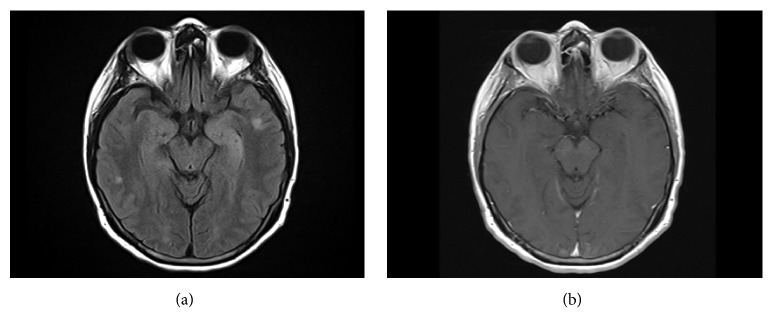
MRI brain imaging for Case*    *2. (a) MRI brain FLAIR imaging. This image demonstrates mildly expansile T2 signal hyperintensity of the left greater than right mesial temporal lobes. Additional small regions of cortical and subcortical T2 signal hyperintensity are noted in the temporal lobes of both hemispheres. (b) MRI brain, T1 sequence with contrast. There is no enhancement noted in the affected areas after administration of gadolinium contrast.
